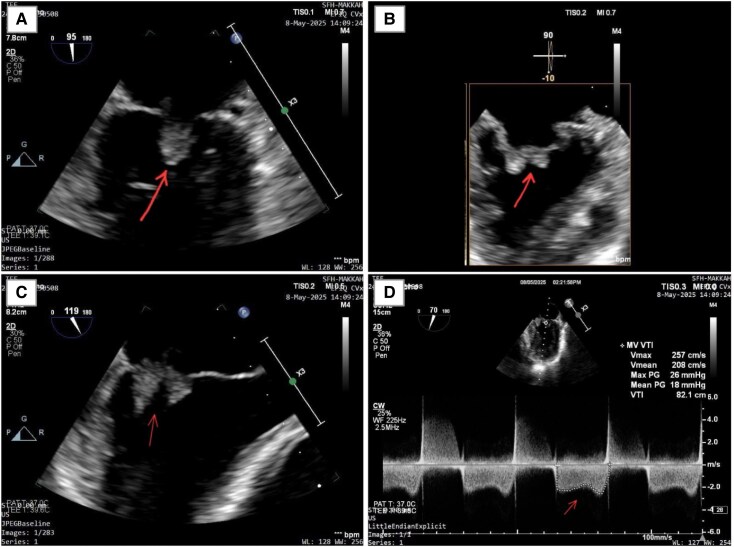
Transoesophageal echocardiography revealed; (*A*) and (*B*) 2 chambers view revealing large mitral valve masses attached to the tips of the mitral leaflets and on the A1/P1 segments, measuring approximately 11–12 mm by 13–15 mm. These masses giving a ‘kissing’ appearance. (*C*) TEE Long axis 3 champers view. (*D*) 2 chambers view with a Continuous Wave (CW) Doppler on mitral valve revealing mean gradient 18 mmHg (severe mitral stenosis), and Mitral regurgitation +2.
